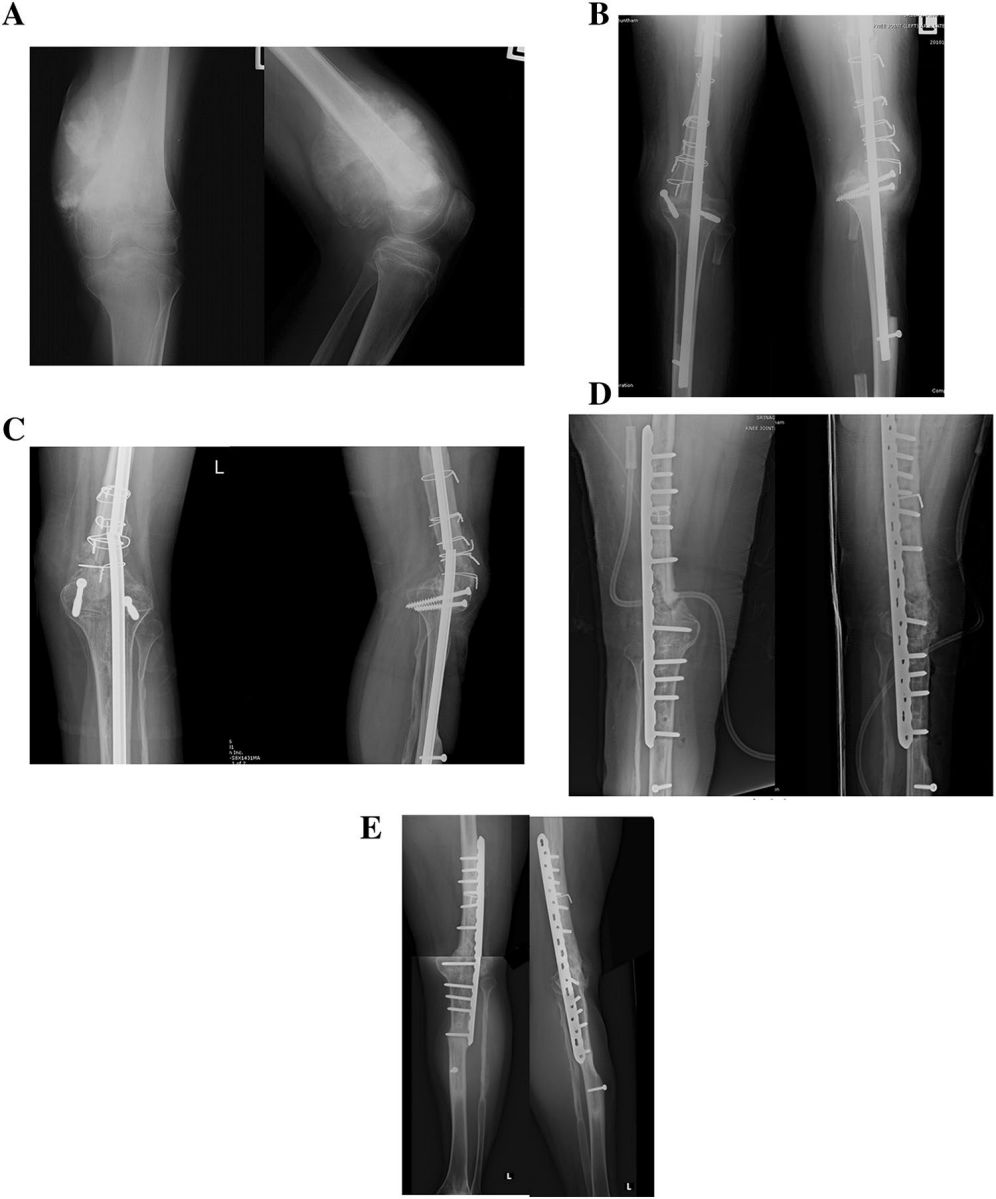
Thirteen-year-old male with osteosarcoma of the distal femur with extensive muscle involvement. (A) preoperative anteroposterior and lateral radiograph. (B) Postoperative radiograph after wide resection and reconstruction with tibial turn-up and augmentation with nonvascularized fibula graft (resection arthrodesis). (C) Break of intramedullary nail occurred 2 years postoperatively. (D) Revision surgery with plate and screws. (E) Eight years after the index procedure with bone union.
